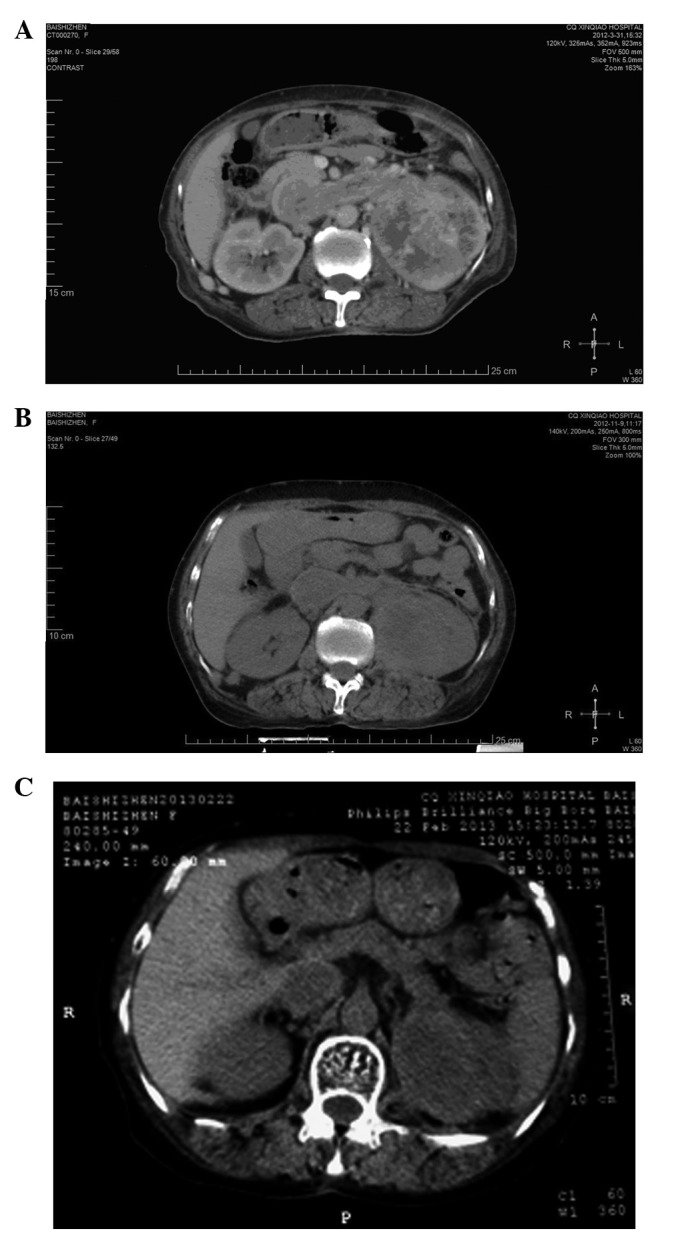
(A) Contrast enhanced computerized tomography (CT) scan shows a mass on the left kidney, and malignant thrombosis of the renal vein and inferior vena cava prior to radiation therapy. (B) The CT scan at the eight- and (C) 11-month follow-up.
